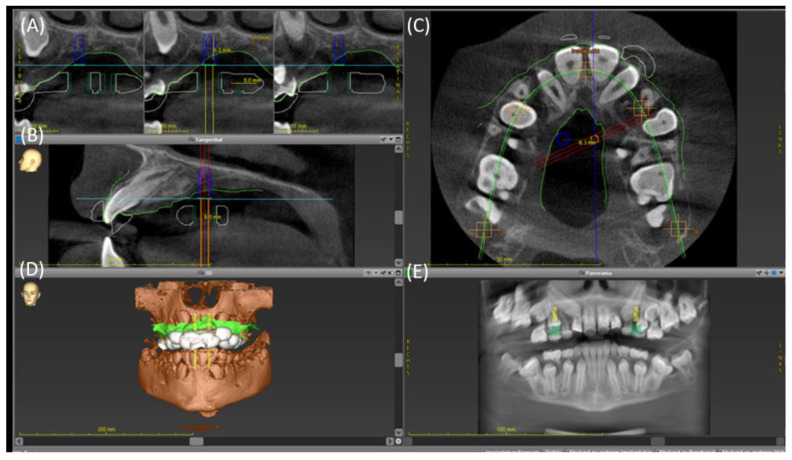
Screenshot of the planning of the two palatal mini-implants using the coDiagnostiX^TM^ (Dental Wings GmbH, Chemnitz, Germany). (A) The planned implant position in the coronal plane showing the vertical bone dimension of the hard palate. (B) Sagittal plane of the right implant ensuring a sufficient distance to the root of the inclined lateral incisor. (C) Transversal plane of the maxilla displaying the multiple retained tooth germs restricting the region for safe mini-implant insertion. (D) Three-dimensional depiction of the bone (brown), the intraoral maxillary soft tissue scan (green), and the final design of the drilling template (white). (E) Panoramic radiograph-like view of the case displaying the planned mini-implant positions.
